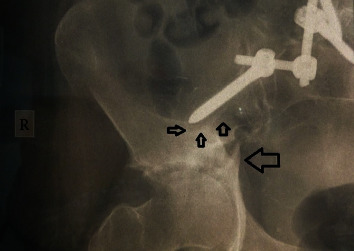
Anteroposterior roentgenogram of the right hemipelvis 18 months postoperatively showing a transverse-type Letournel fracture of the right acetabulum (big arrow). Small arrows show the radiolucent area around the tip of the right iliac screw. There is radiolucency around the iliac screw.
